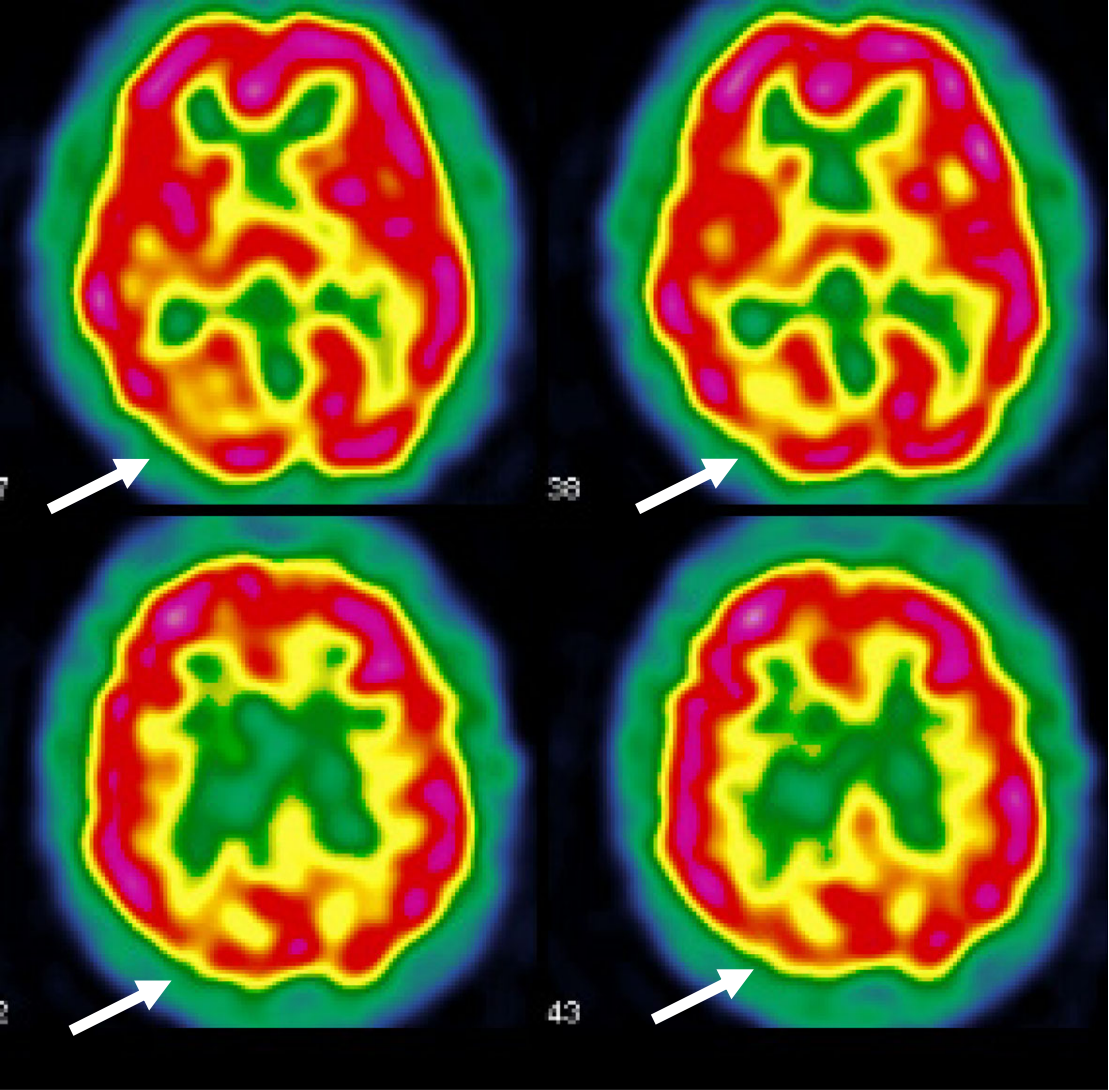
Second cerebral SPECT showing hypoperfusion in the right temporo-occipital junction, right inferior parietal lobe and left superior frontal lobe
